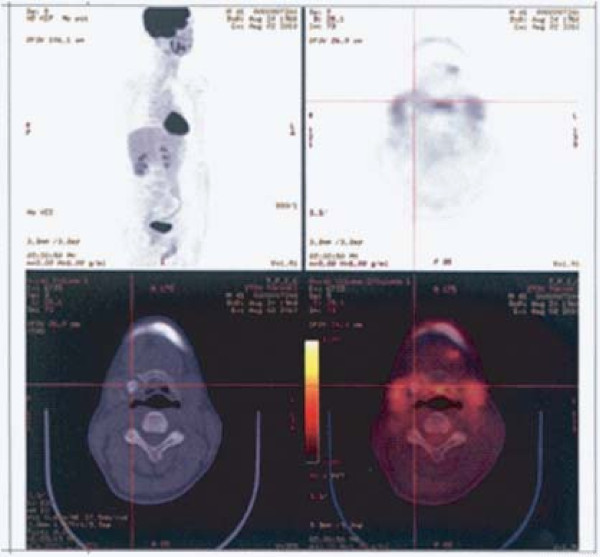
Positron emission tomography-computed tomography. No abnormal accumulation in the hyoid tumor or systemic abnormalities were evident.
